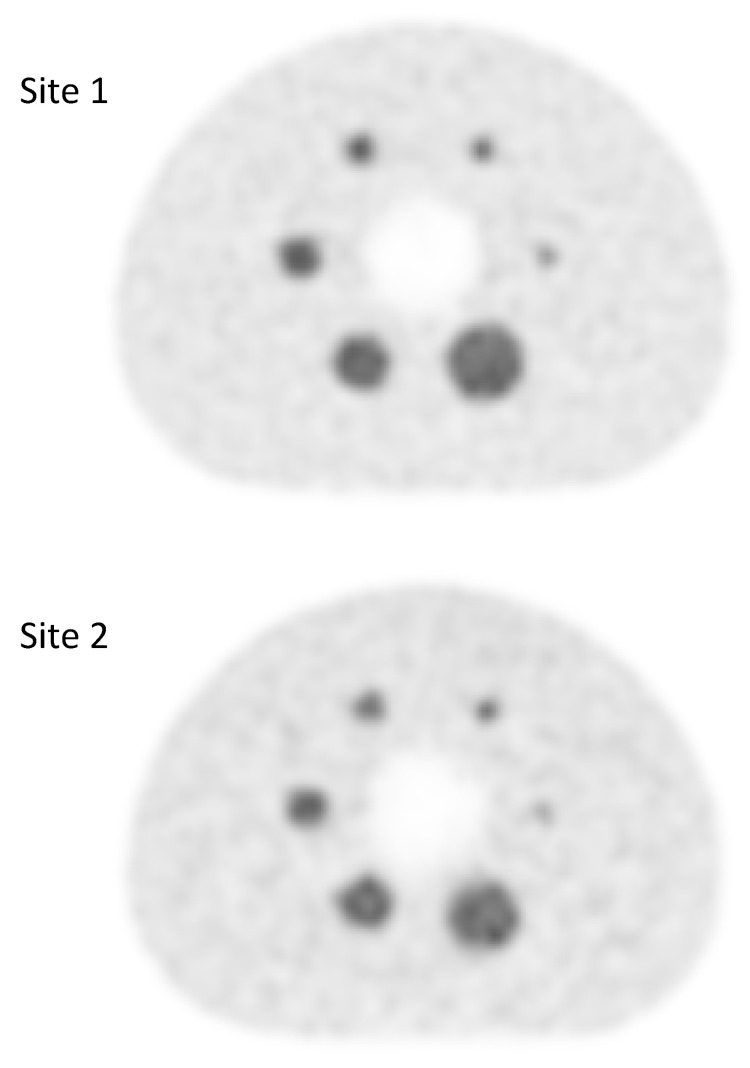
Qualitative assessment of FDG PET images obtained at site 1 (GE Discovery 690 PET scanner) and at site 2 (Siemens Biograph mCT 64 PET scanner).
